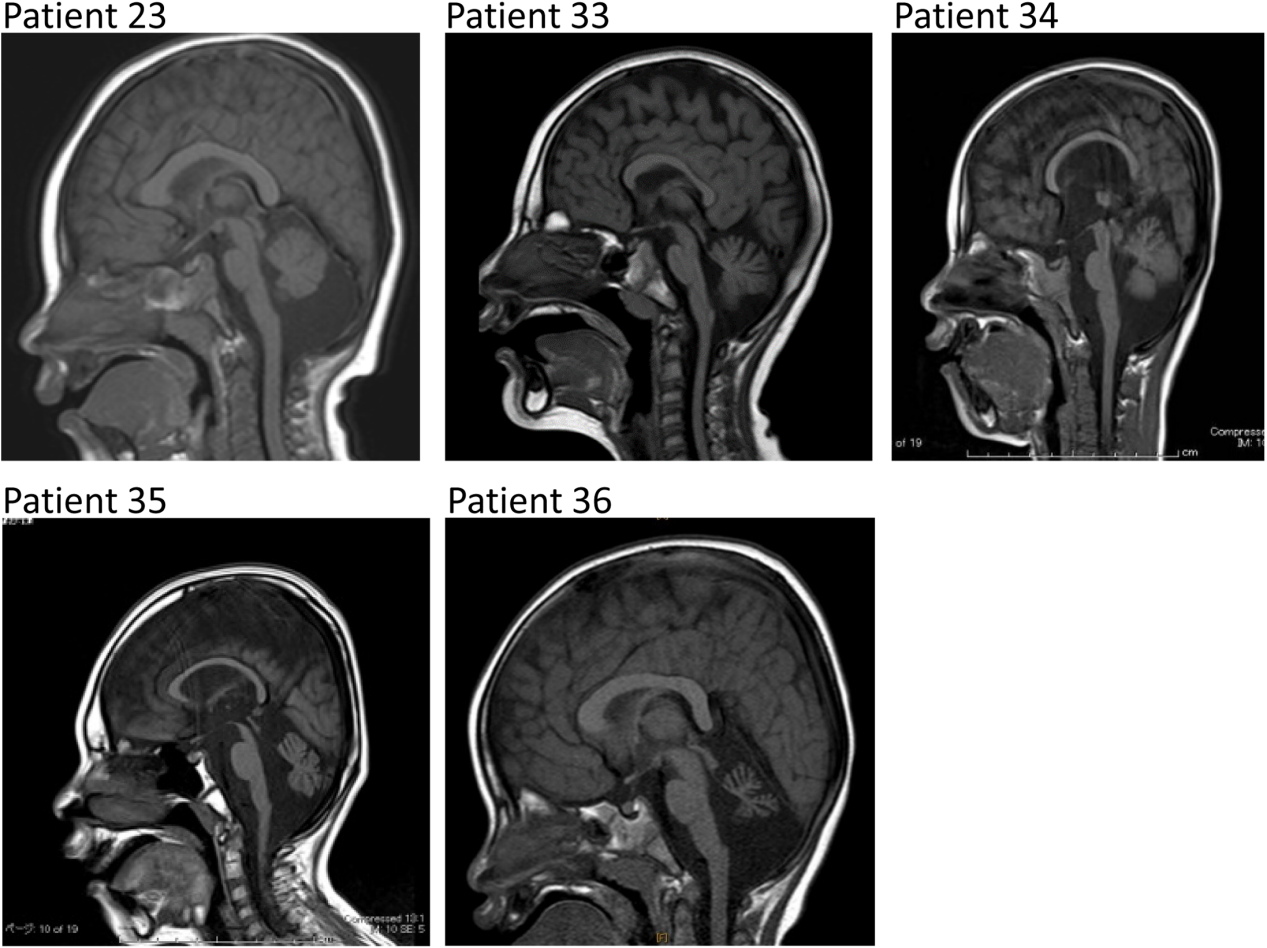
Representative sagittal images of brain MRI scans of five patients. Regardless of the distinct etiologies, all patients show features resembling MICPCH.
